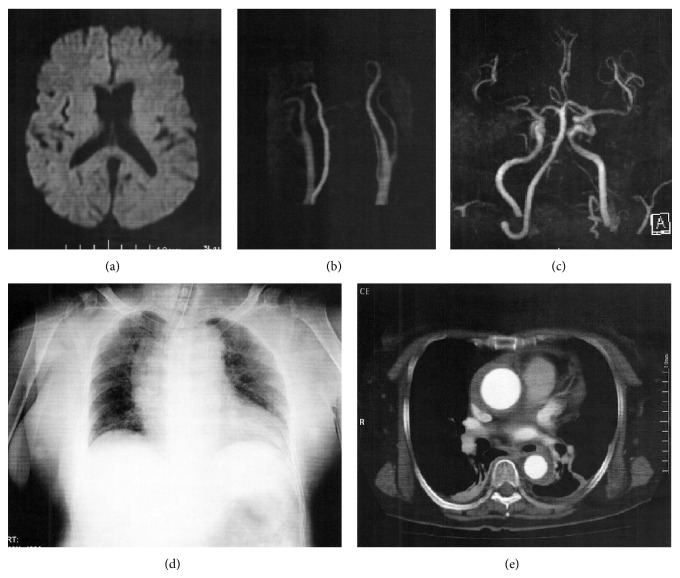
Axial head MRI-DWI (a), carotid MR angiography (b), head MR angiography (c), chest radiography (d), and axial chest to abdominal enhanced CT (e). Her pseudolumen had already been thrombosed and closed.
